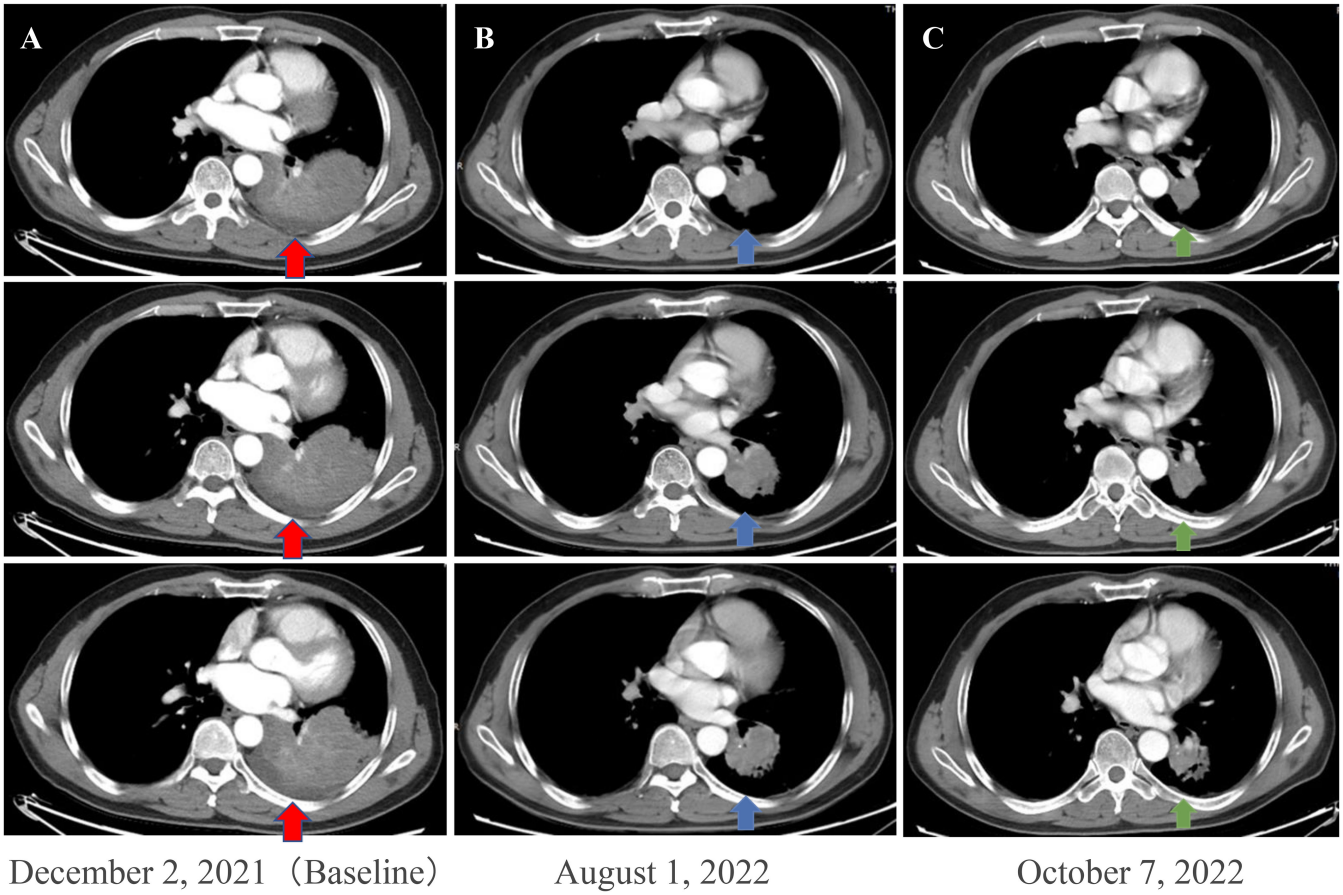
Enhanced chest CT scans of the patient during neoadjuvant therapy. (A) Baseline imaging demonstrating a 93 mm × 70 mm abnormal lung mass (red arrows) in the lower lobe of the left lung. (B) After 210 days of osimertinib therapy, the chest CT scan shows mass shrinkage (blue arrows) to 48 × 44 mm, achieving a partial response (PR). (C) After two cycles (42 days) of osimertinib and chemotherapy, the chest CT scan shows mass shrinkage (green arrows) to 40 mm × 37 mm, achieving a sustained partial response (sPR). CT, computed tomography.
